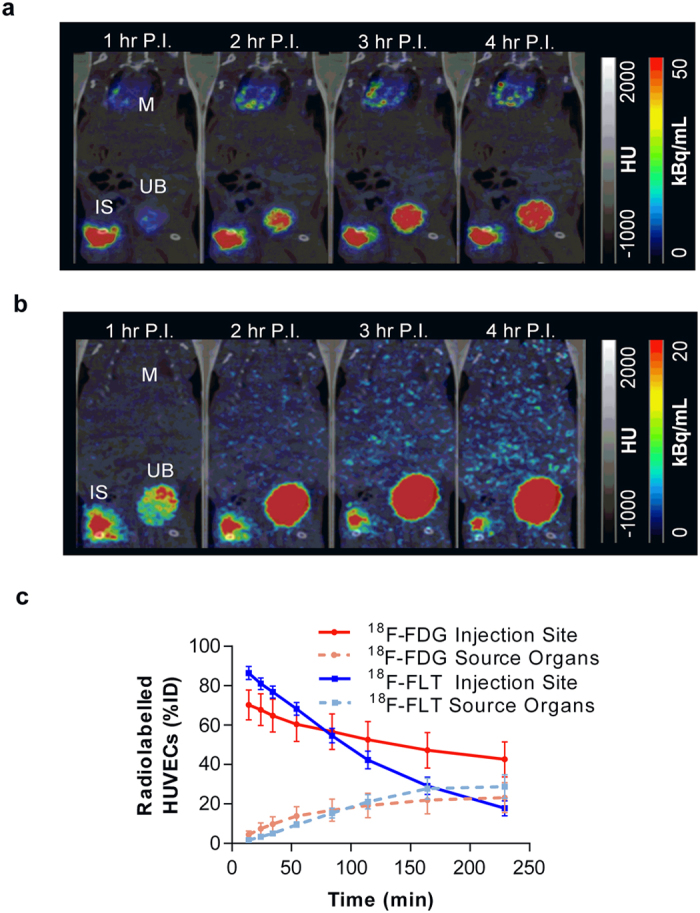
Comparison of ^18^F-FDG and ^18^F-FLT-labelled cell signal profiles. Representative averaged (1 hr) images from each hour post-injection (P.I.) of (a) ^18^F-FDG- and (b) ^18^F-FLT-labelled HUVECs. IS = injection site, M = myocardium and UB = urinary bladder. (c) ^18^F-FDG- and ^18^F-FLT-labelled HUVECs time activity curves at the injection site and source organs, n = 3.
